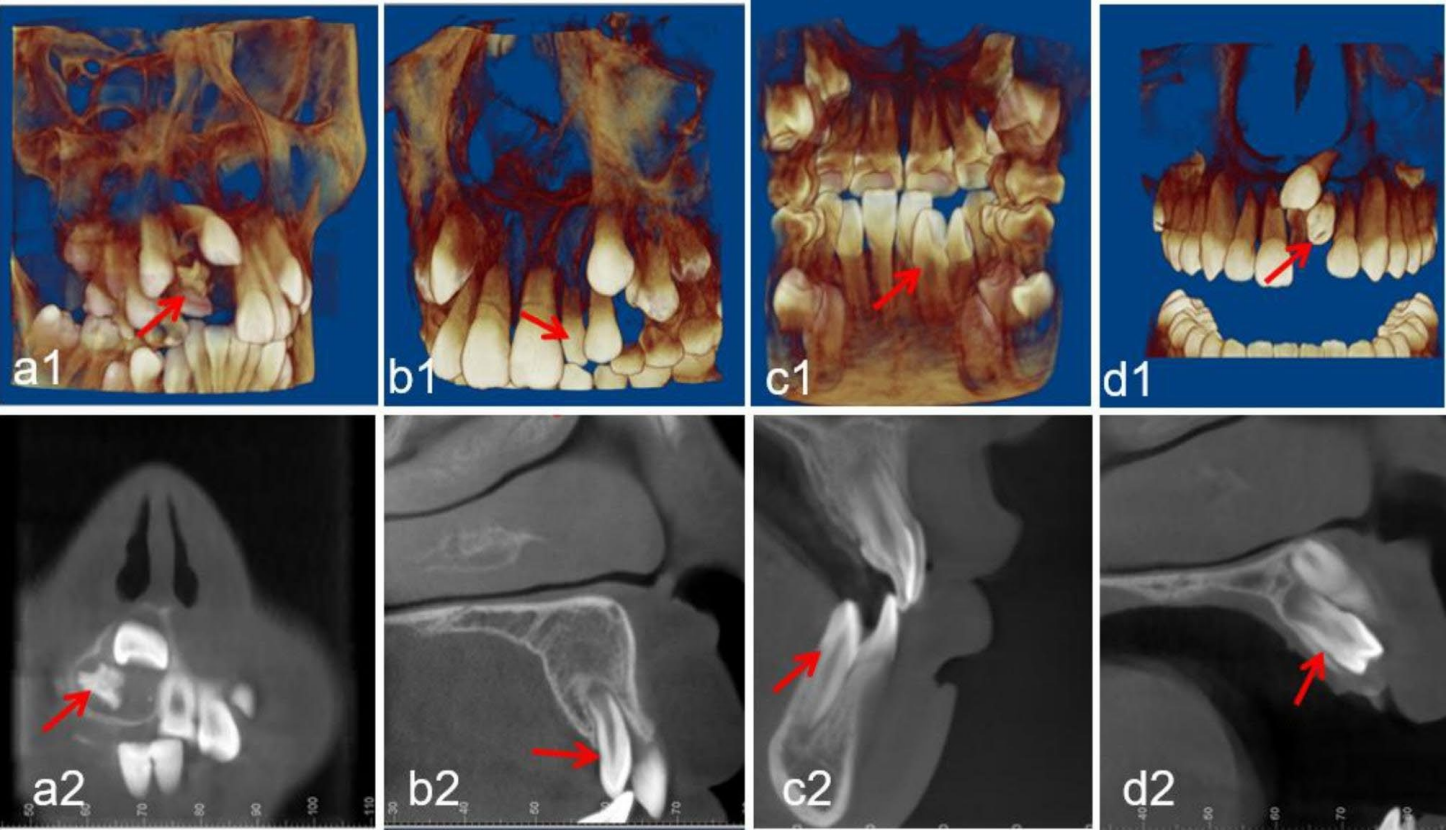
Representative CBCT images exhibiting diverse morphology of ST. (a1-a2) odontoid morphology; (b1-b2) conical morphology; (c1-c2) supplemental morphology of lateral incisor; (d1-d2) tuberculate morphology. The red arrows point to the ST in the CBCT images
